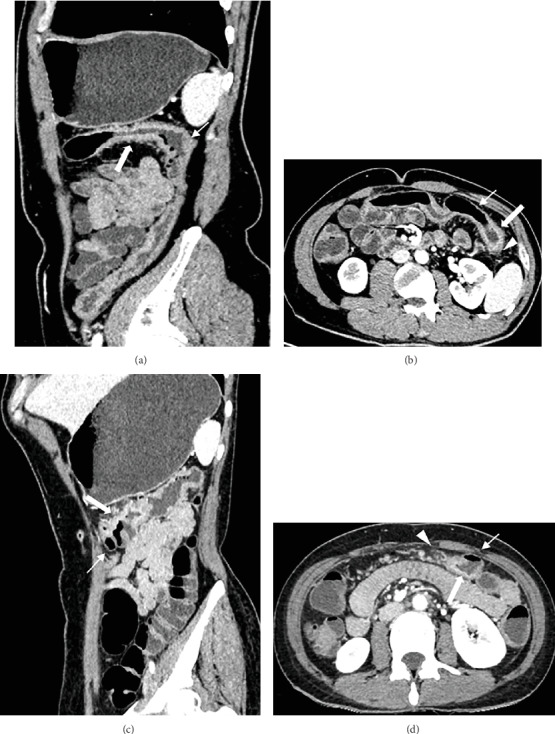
CT enterograph features of case 1 (a, b) and case 3 (c, d): asymmetric thickening (small arrow), layered attenuation (big arrow), fat stranding (triangle).
